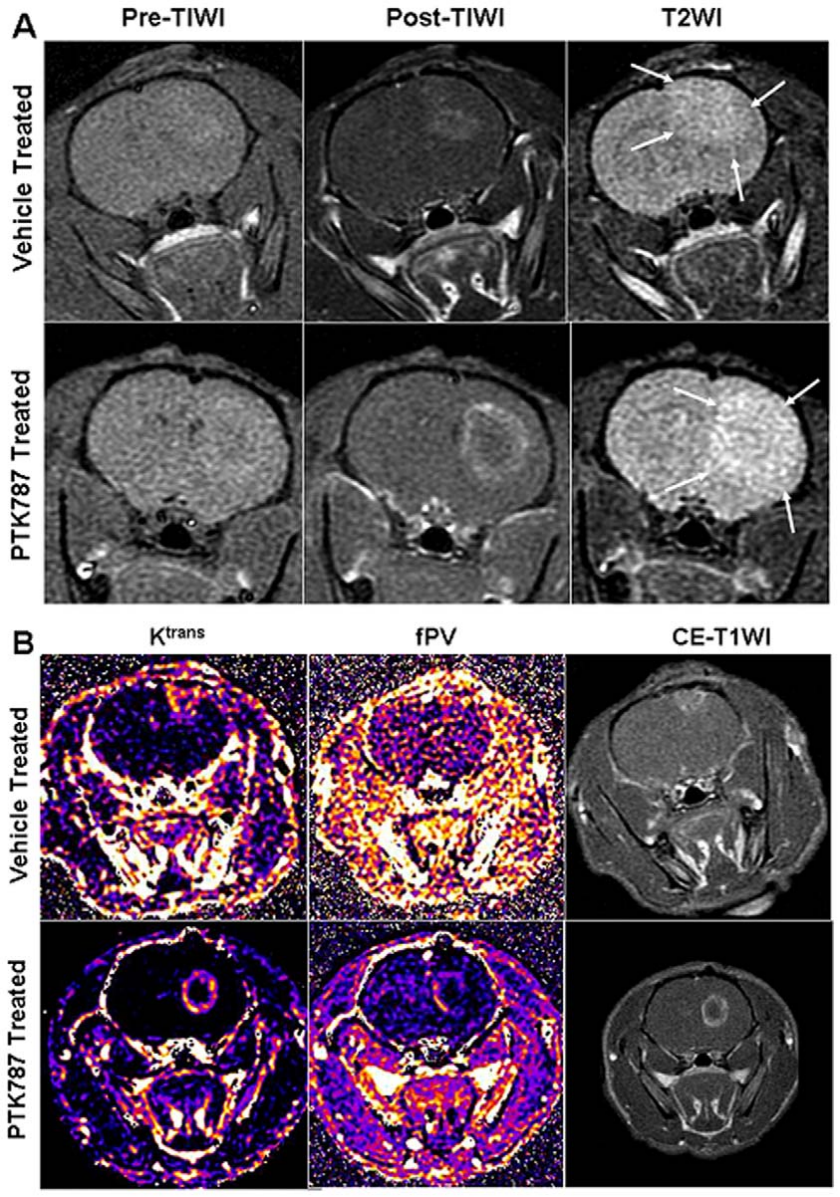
MRI and parametric analysis of PTK787 treated and vehicle treated tumors. (A) Representative cases from vehicle and PTK787 treated tumors show enhancement following administration of contrast agents (Post T1WI) and changes in T2WI. PTK787 treated tumor show larger tumor with prominent rim enhancement and central hollow compared to that of vehicle treated tumor. T2WI also show larger area of high signal intensity beyond the margin of enhancement (arrows). (B) Representative cases from vehicle and PTK787 treated tumors showing K^trans^, fractional plasma volume (fPV) and corresponding post contrast T1W images. Clearly increased K^trans^ and fPV at the periphery of PTK787 treated tumor (calculated K^trans^ and fPV maps are adjusted to same scale level for both vehicle treated and PTK787 treated tumors) indicate higher permeability and fPV, which are in agreement with the findings of dilated vessels at the peripheral part of PTK787 treated tumors (see below).
